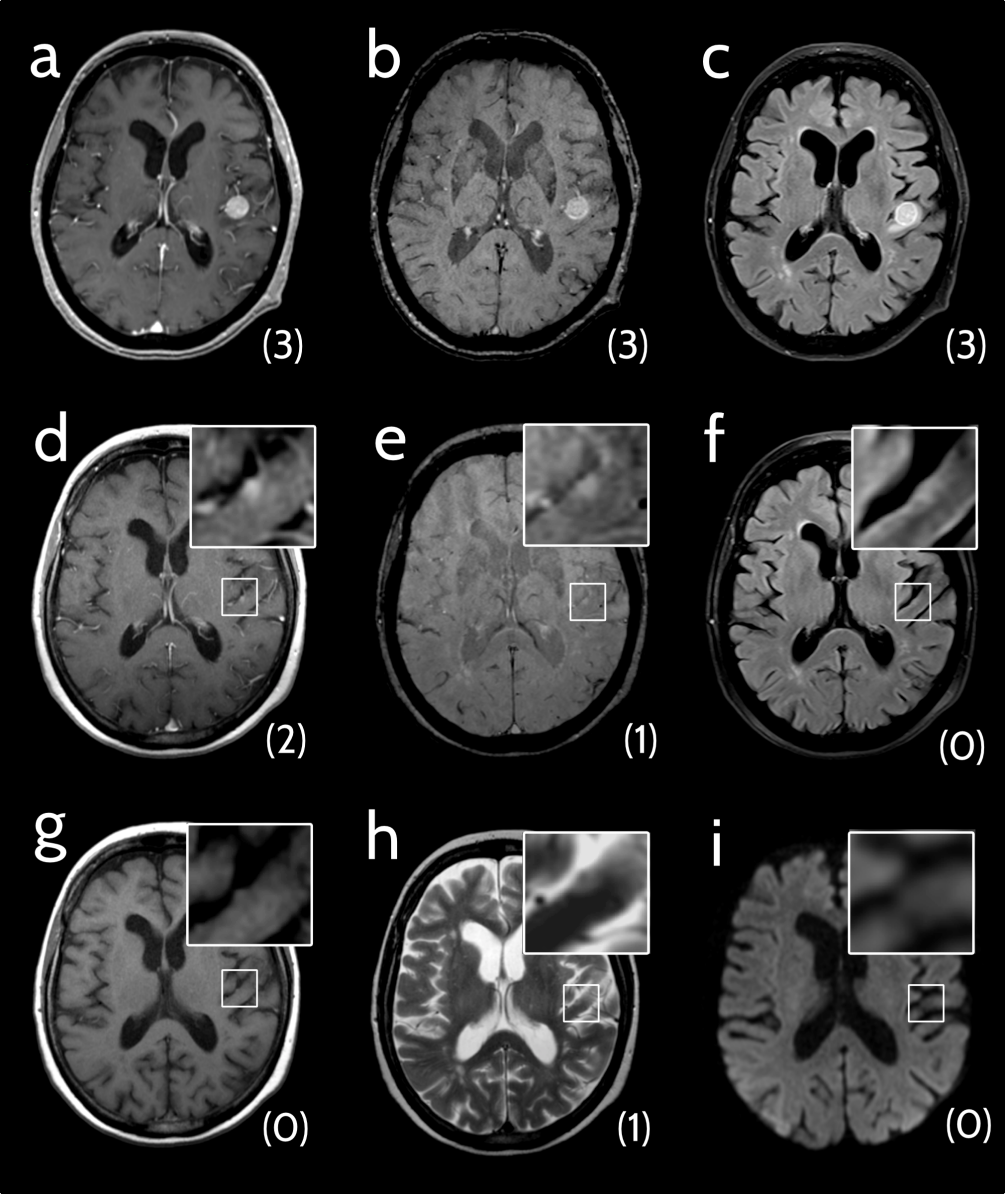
Analysis of pre-diagnosis cMRIs. 69-year-old woman with a melanoma brain metastasis (MBM) that was detectable in an examination prior to the one that led to diagnosis. Conspicuity scores are indicated in brackets below the lesions. (a-c) Axial cMRI scans of a patient with newly diagnosed MBM. (a) Contrast-enhanced T1-weighted image (ceT1w): conspicuity score (CS) 3. (b) Susceptibility-weighted image (SWI), CS 3. (c) Fluid-attenuated inversion recovery (FLAIR) image: CS 3. (d-i) Axial cMRI scans of the pre-examination (ca. 9 weeks earlier).(d) CeT1w: CS 2. (e) SWI: CS 1. (f) FLAIR: CS 0. (g) Non-enhanced T1-weighted image: CS 0. (h) T2-weighted image: CS 1. (i) Diffusion-weighted image (TRACE): CS 0.
